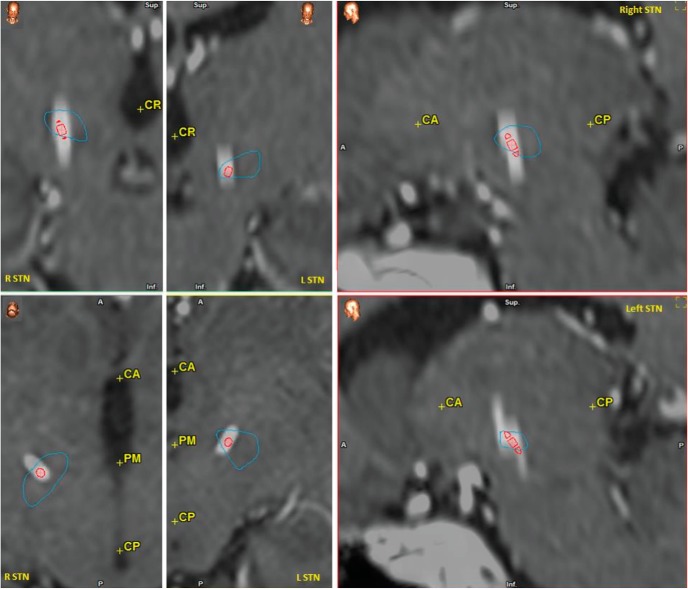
2D reconstruction of recording coordinates. Location of STN (pale blue lines) and of Medtronic 3389 DBS contact 1 in GDP #6 (already shown in Fig. 1*D*). R, right; L, left; CA, anterior commissure; CP, posterior commissure; PM, middle point between CA and CP line; CO, MRI coronal view; SW, MRI sagittal view; AX, MRI axial (transverse) view; Sup, superior; Inf, inferior; A, anterior; P, posterior.
